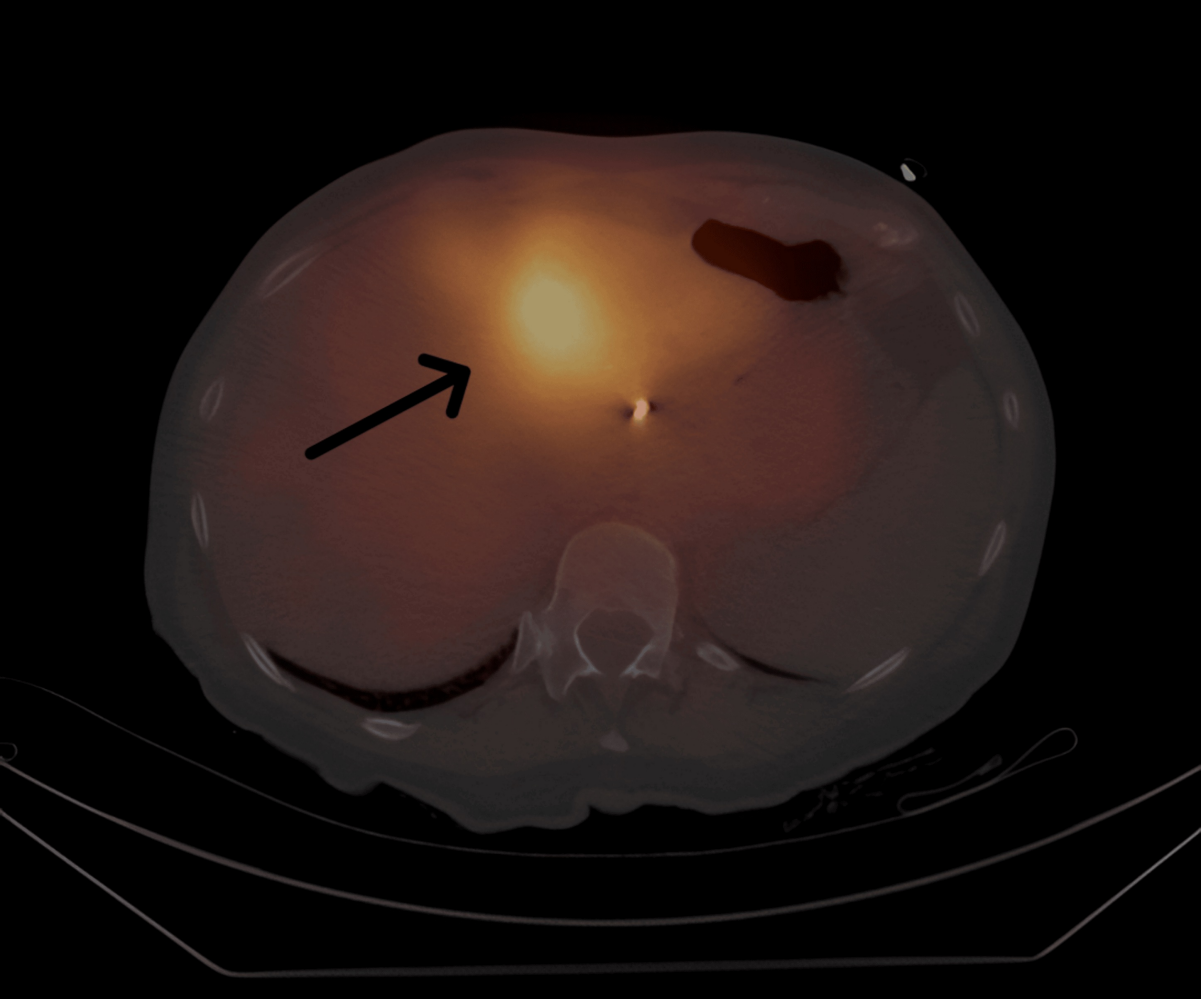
A post-therapy yttrium-90 (Y-90) Bremsstrahlung single-photon emission computed tomography (SPECT) scan. Post-treatment Bremsstrahlung SPECT imaging demonstrating tracer distribution localized to the left hepatic lobe without significant extrahepatic uptake, confirming appropriate yttrium-90 microsphere delivery (black arrowhead).
